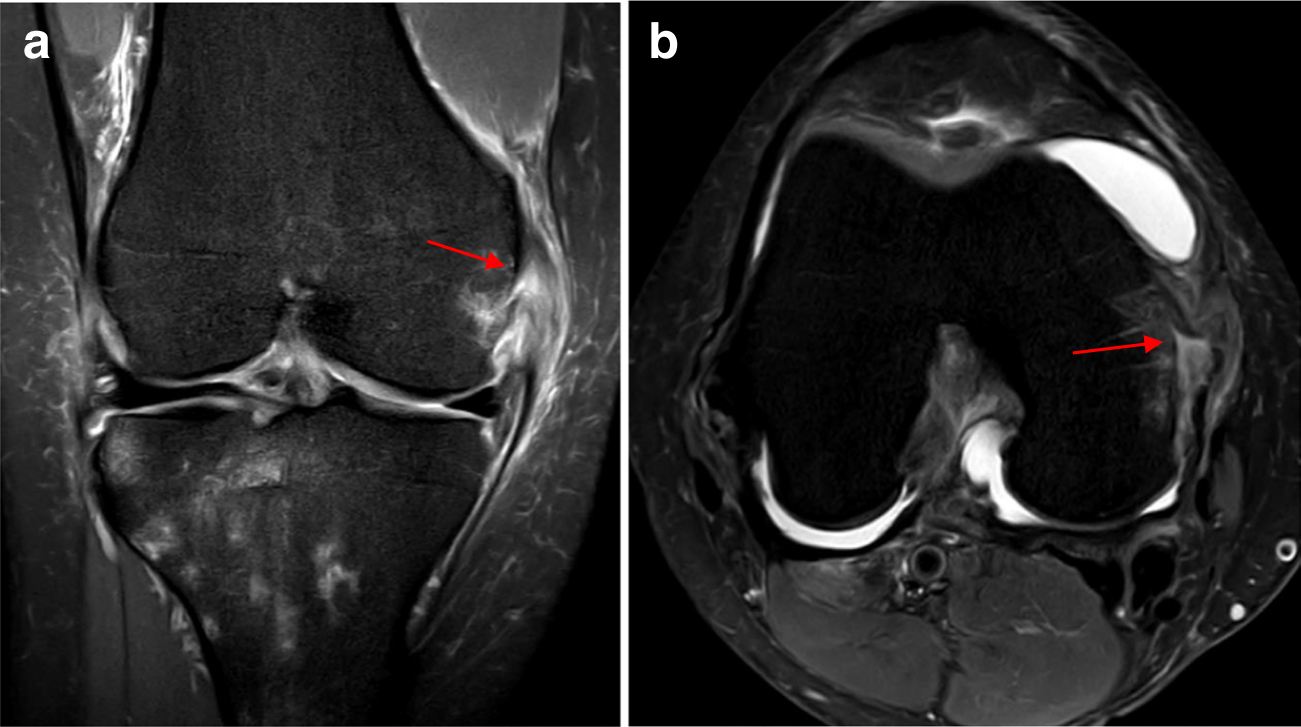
Complete proximal medial collateral ligament tear (superficial and deep) (arrows) on coronal (a) and axial (b) proton density fat saturation magnetic resonance imaging sequences (right knee)
